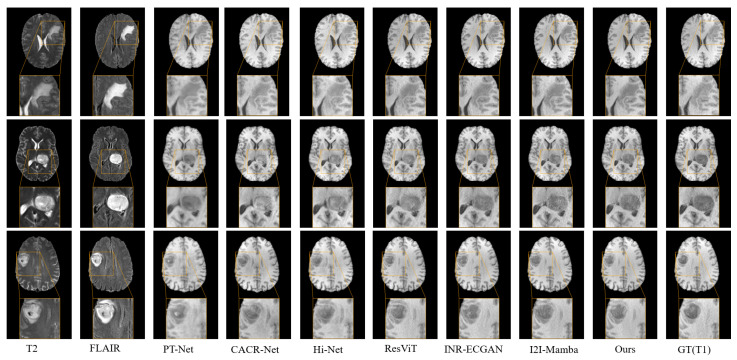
Visual comparison of the proposed HMF-MambaINR with other methods on the synthetic T1 task (T2, FLAIR → T1) using the BraTS 2020 dataset.
